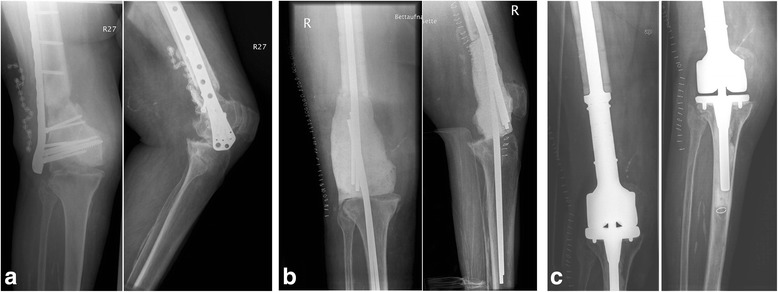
Anterior-posterior and lateral X-rays of the right knee with thigh of a 74-year-old woman with a infected pseudoarthrosis of the right distal femur after plate osteosynthesis of a supracondylar femur fracture b resection of the distal femur and implantation of an intramedullary cement spacer c after implantation of a silver- coated distal femoral replacement endoprosthesis (System MUTARS® Modular Universal Tumor And Revision System, Implantcast GmbH, Buxtehude, Germany)
